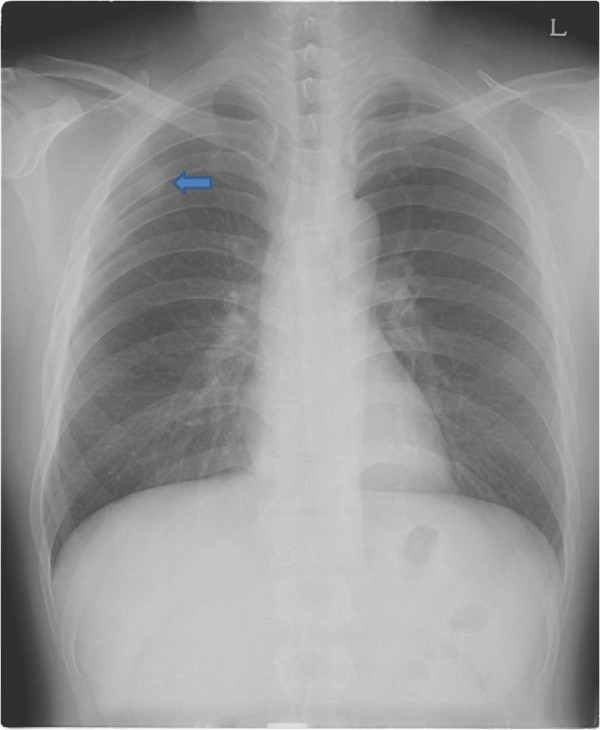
Preoperative chest radiograph (PA) view shows a round opacity on the right upper lung field (arrow).
